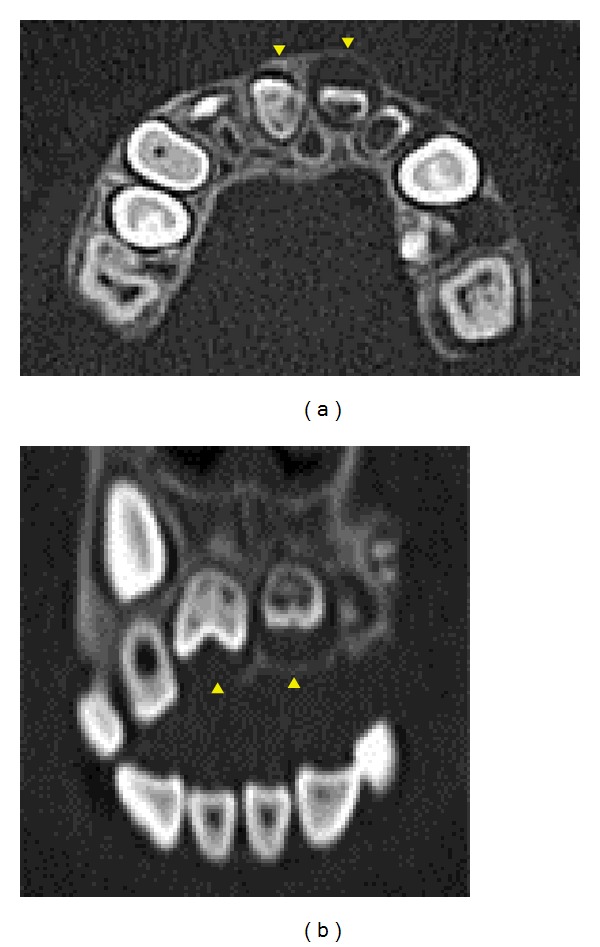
CT images of case 1. (a) Axial CT image shows labial expansion of dental follicles (arrowheads). (b) Multiplanar reconstruction image shows dental follicles clearly expanding toward alveolar ridge (arrowheads).
